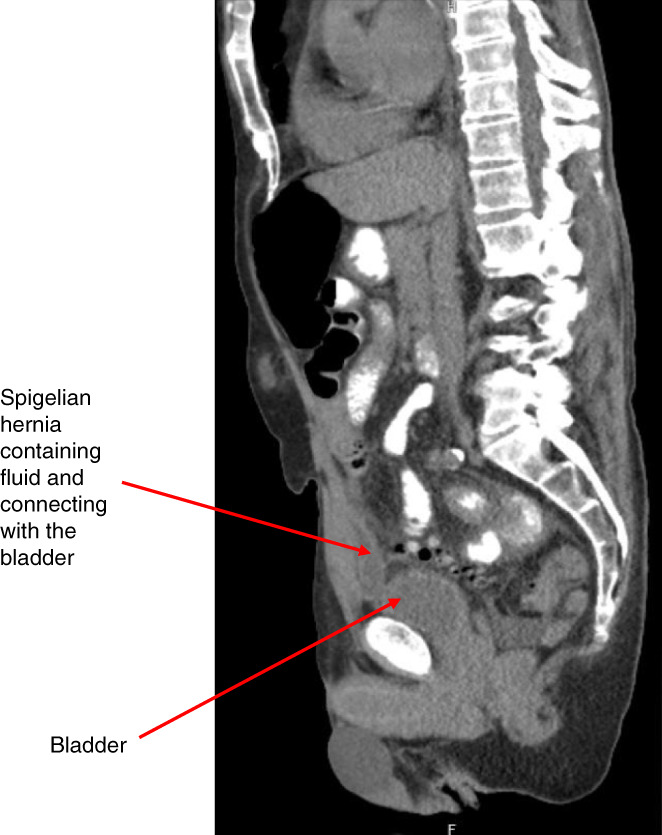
CT of the abdomen without contrast. CT, computerized tomography.
